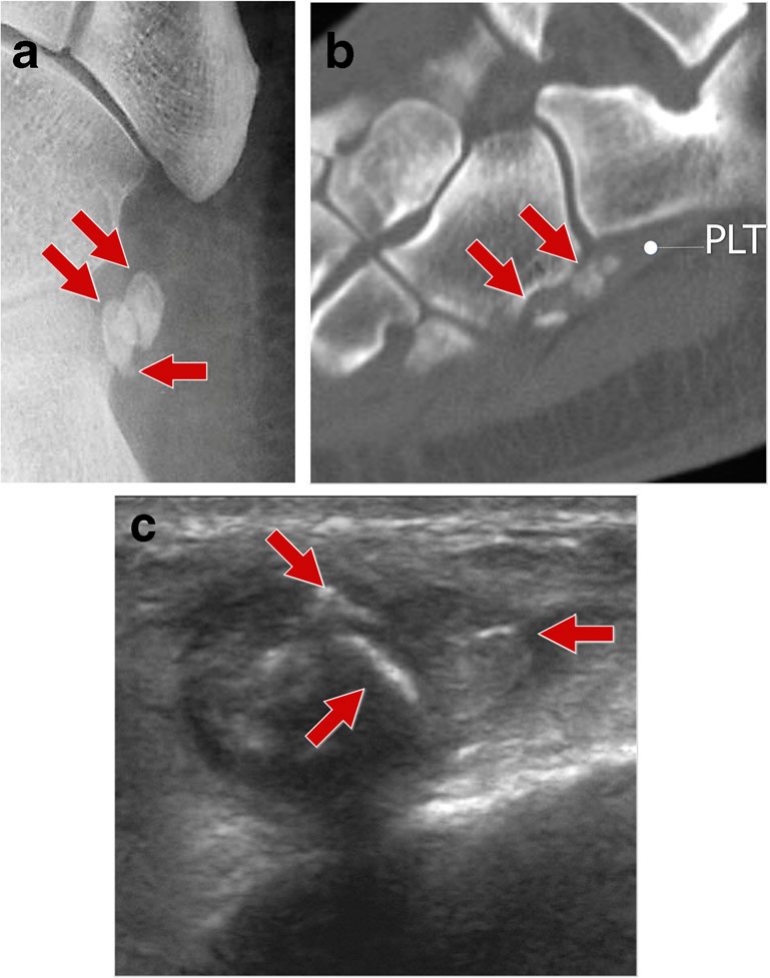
Multipartite os peroneum (*arrows*; different patients). *3A* Standard radiograph. *3B–C* Computed tomography and ultrasound. Accurate evaluation of the bone margins is of the utmost importance for differential diagnosis with a fracture. CT is the technique of choice to differentiate a multipartite OP from a non-displaced fracture. *PLT* peroneus longus tendon
